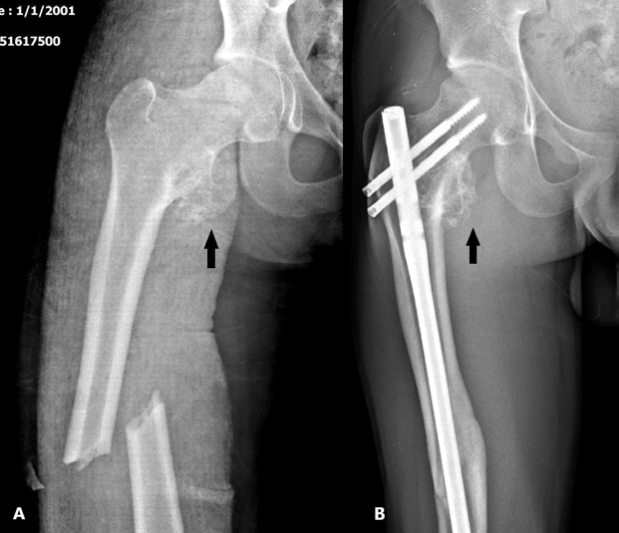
The dislocated fracture line in the right femoral diaphysis on AP X-ray radiography of a 19-year-old male case (A). Six months after the treatment, a healed fracture line and metallic operation material are observed (B). *Osteochondroma* localized at the level of the right *lesser trochanter* (shown with black arrows) on both X-rays.
